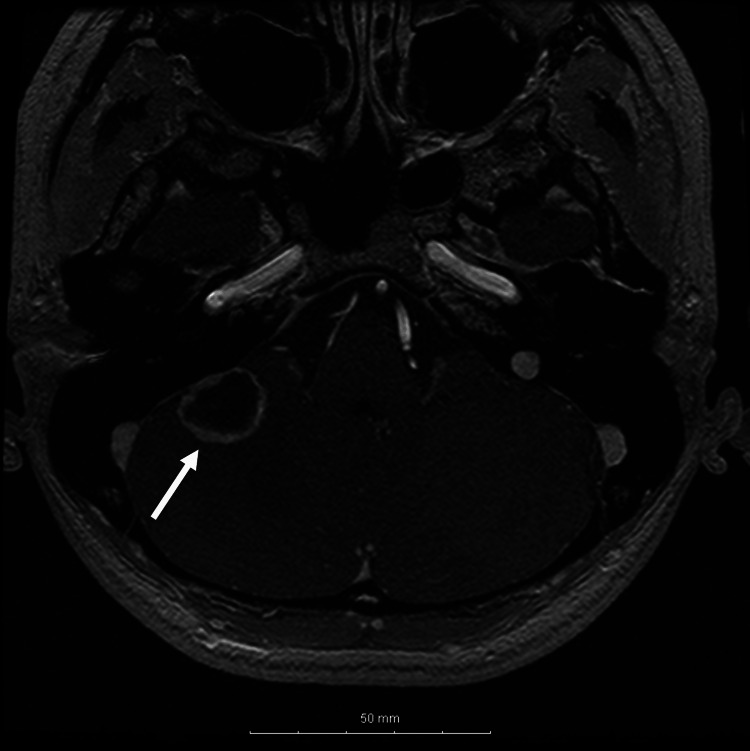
Magnetic resonance imaging of the brain performed six months before pregnancy A hemangioblastoma in the right cerebellum is visible on a T1-weighted image with gadolinium-based contrast.
